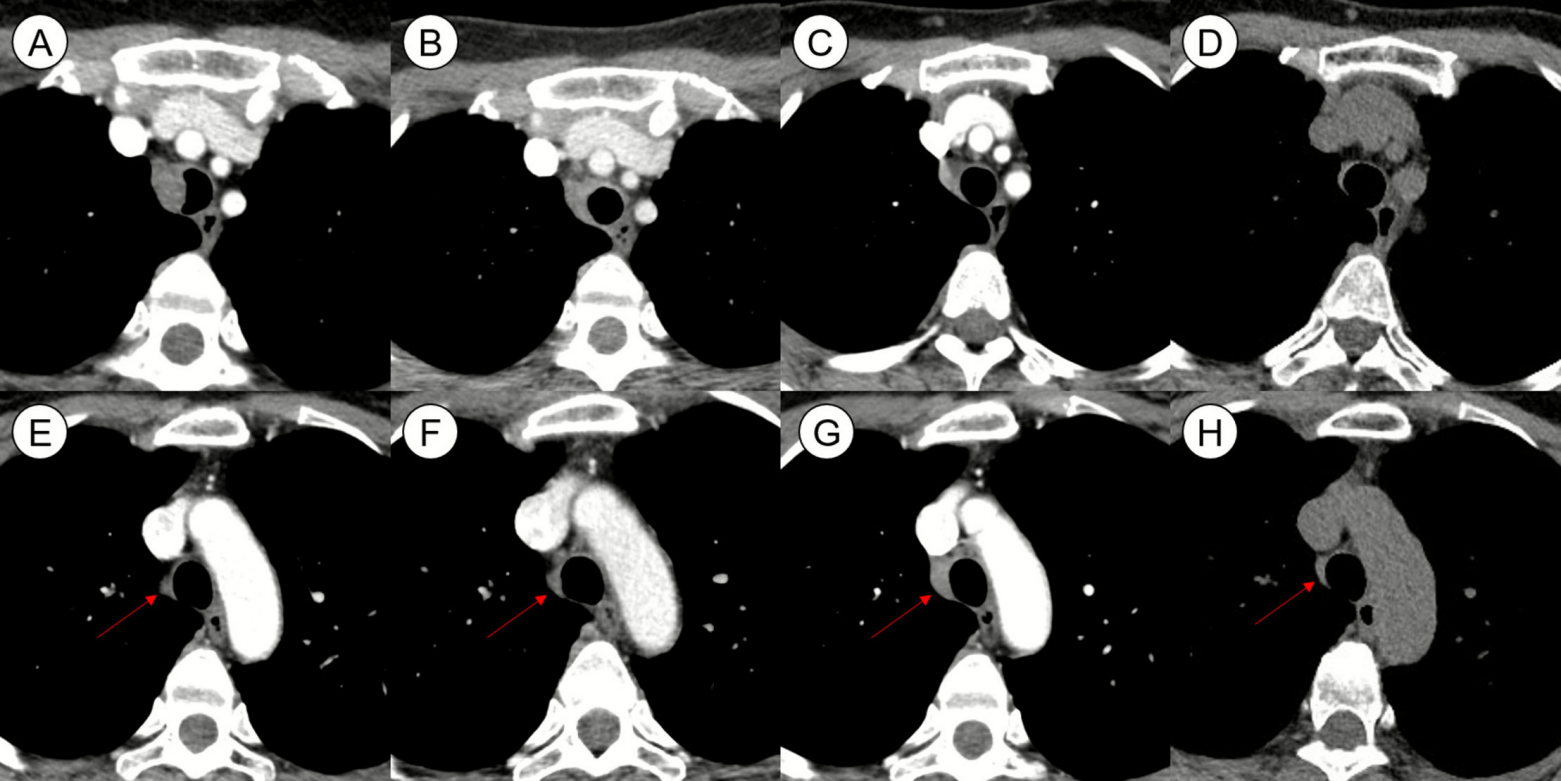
Serial chest computed tomography (CT) images of a patient with Rosai–Dorfman disease (RDD) at the T4 vertebral level. The upper row (A–D) shows axial images at the upper T4 level, and the lower row (E–H) corresponds to lower T4 to upper T5 levels. Red arrows indicate the extraluminal portion of the tumour extending beyond the tracheal wall. (A, E) Initial chest CT performed during a routine health check‐up in a 45‐year‐old woman revealed a 14 mm endotracheal nodule involving the right lateral wall of the upper trachea without prominent extraluminal component. (B, F) Two weeks after bronchoscopic debulking, the endobronchial portion had significantly decreased without progression of the extraluminal component. (C, G) At 20‐month follow‐up post‐debulking, no recurrence of the endotracheal lesion was observed. However, interval growth of the extraluminal component was noted. (D, H) Post‐steroid therapy (4 years), the extraluminal lesion showed a notable reduction in size.
